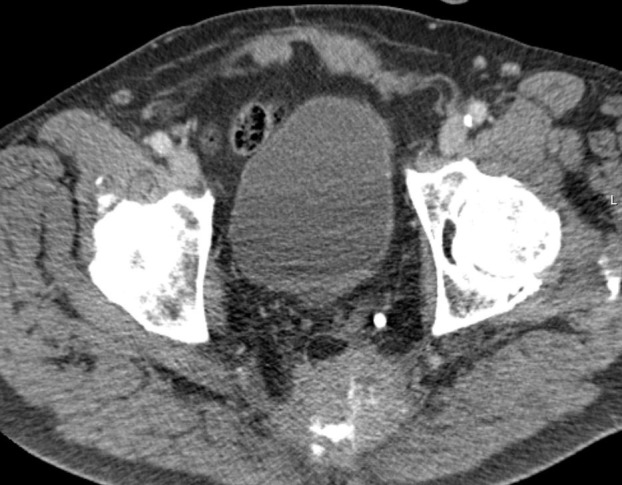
Axial computed tomography (CT) scan Large sacral and pre-sacral mass.
